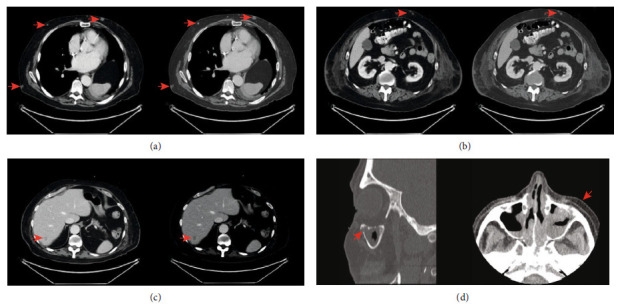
CT scan identified no significant lymphadenopathy but revealed numerous subcutaneous soft tissue nodules throughout the (a) chest and (b) abdominal wall. (c) A low-attenuation hepatic lesion within segment VII of the liver. (d) A sagittal and transverse view of maxillary sinuses shows bilateral mucosal thickening and focal erosion of the anterior wall of the left maxillary sinus with extrasinusoidal extension into the overlying soft tissue. Note: red arrows indicate relevant findings.
